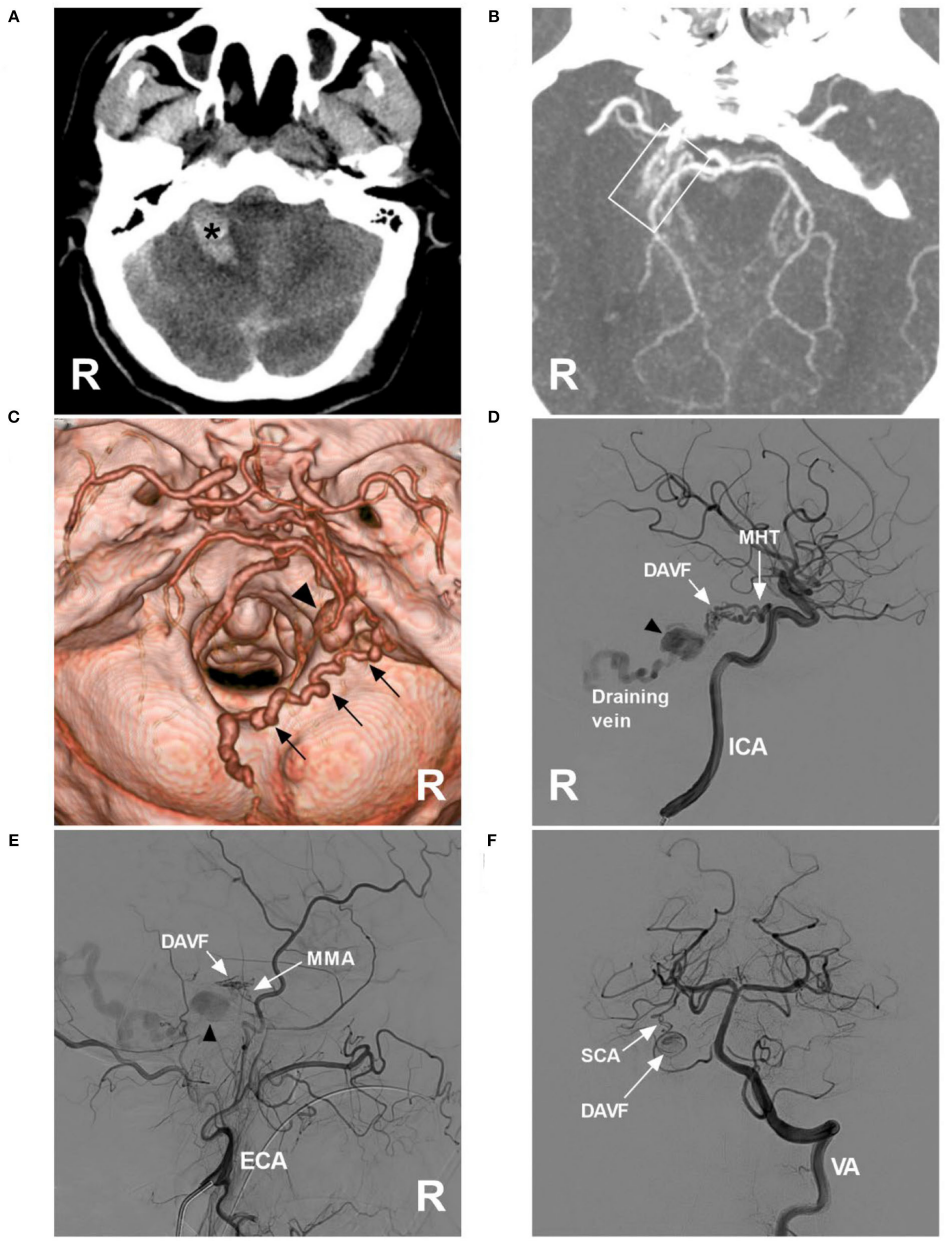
Preoperative images in case 1. (A) CT showing right cerebellar hemorrhage (asterisk). (B) Maximum intensity projection of CTA showing a tangle of abnormal vessels (frame) at the right margin of the tentorium; a DAVF was suspected. (C) Three-dimensional reconstruction of CTA showing a tortuous draining vein (multiple arrows) along the cerebellopontine fissure, the surface of inferior cerebellar hemisphere, and inferior vermin, went into the torcular herophili, with varix (triangle) at the beginning. (D) DSA of the right ICA showing that the MHT was the main feeding artery, and there was varix on the draining vein (triangle). (E) DSA of the right ECA showing that the petrous branch of the MMA was a minor feeding artery, and varix (triangle) was indicated. (F) DSA of the VA showing that the artery of Wollschlaeger and Wollschlaeger of the SCA was the minor feeding artery. CT, computed tomography; CTA, CT angiography, DAVF, dural arteriovenous fistula; DSA, digital subtraction angiography; ECA, external carotid artery; ICA, internal carotid artery; MHT, meningohypophyseal trunk; MMA, middle meningeal artery; R, right; SCA, superior cerebellar artery; VA, vertebral artery.
